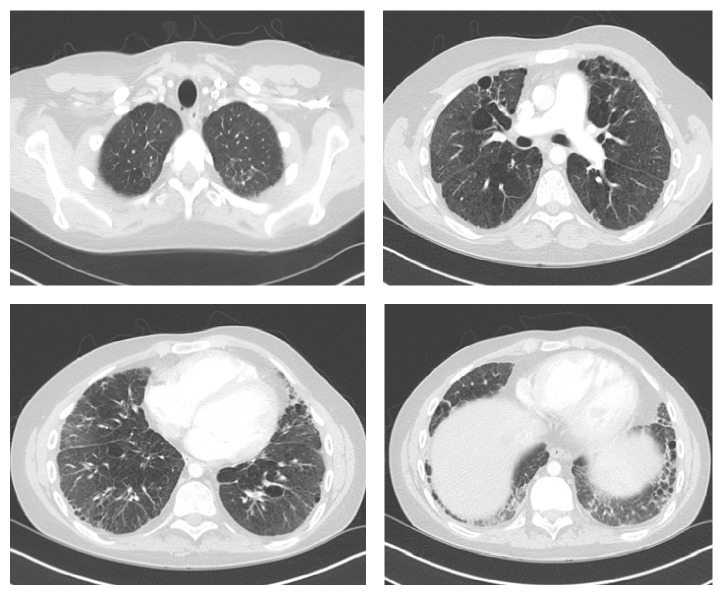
CT of chest showed progressive fibrosis, with increased numbers of lung small cysts, as well as area of emphysema. Additional finding included subpleural honeycombing and mosaic attenuation.
